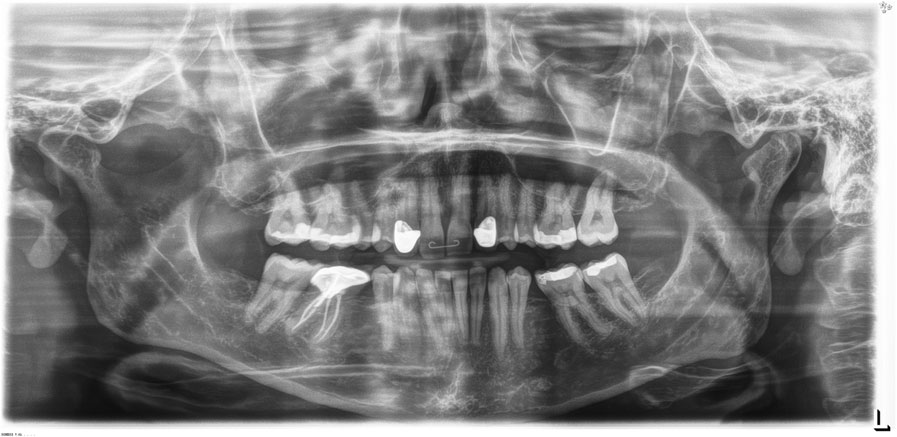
Panoramic Dental Radiograph. Panoramic dental radiograph from our proband with a pathogenic AXIN2 variant. There are 24 teeth. A complete set of adult is 32 teeth. The patient is lacking her upper lateral incisors, bottom second premolars, and 3 third molars (wisdom teeth)
